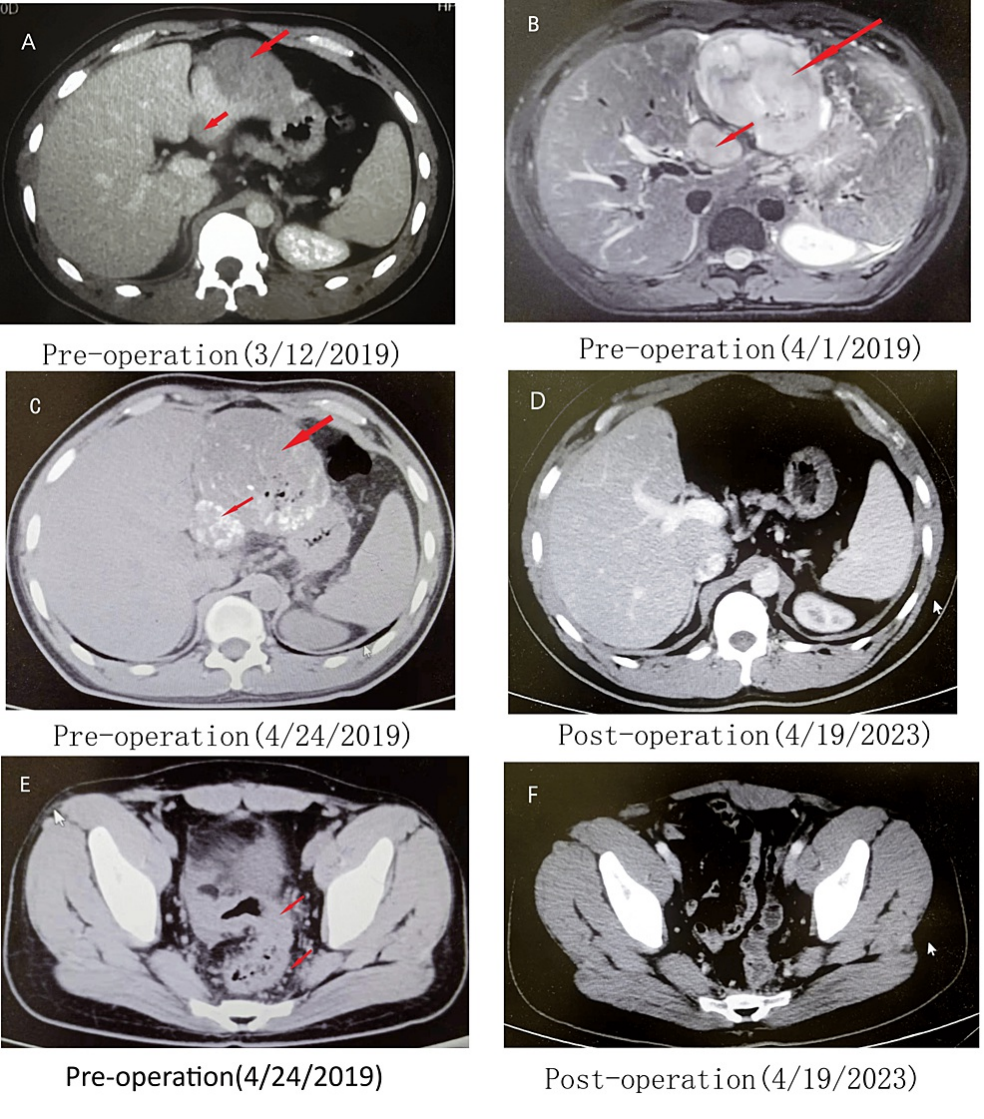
CT and MRI images A: Two metastasis foci in the left liver lobe in the CT image. B: The metastasis foci in the MRI image three weeks later. C: Post-HAIC treatment, metastasis foci in the CT scan before operation. D: The liver CT scan at four years post operation. E: There were lots of lymph nodes in the rectal mesenteric in the pelvic CT pre operation. F: The pelvic CT scan at four years post operation.
